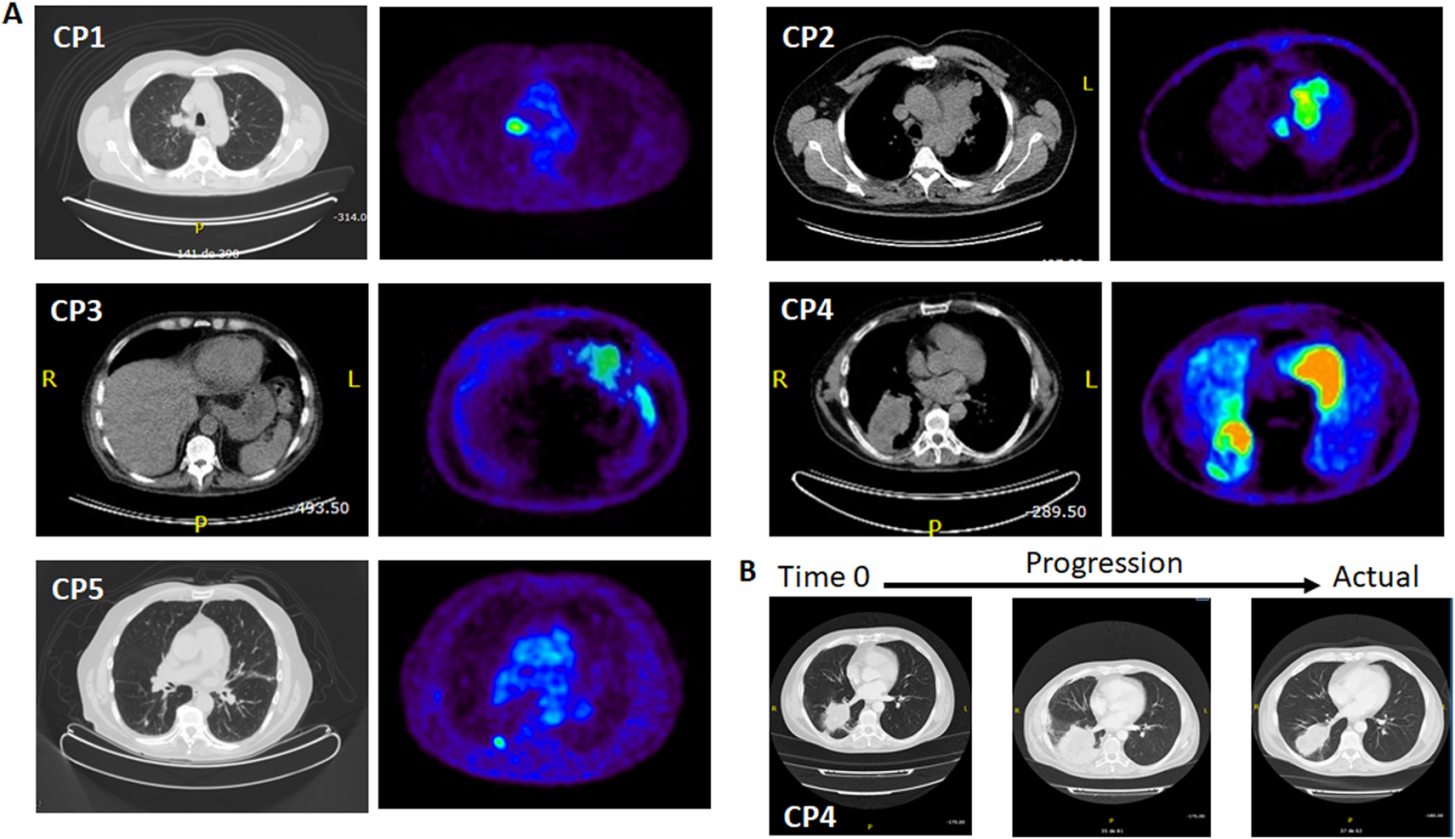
Computed tomography Positron emission tomography scanners. a Cross-sectional CT and PET-CT scans corresponding to the maximum size of the primary tumor from five patients. All patients are in stage IV (metastatic) but with different extensions and size of the primary tumor. CP1, adenocarcinoma patient with a non-bulky primary tumor, but with a very wide metastatic extension (subcutaneous); CP2 and CP3, CA patients with large primary tumors (bulky) but with limited extensions of metastasis; CP4, a priori, patient with a large primary tumor (bulky) with limited extension of metastasis (b); CP5, patient with a non-bulky primary tumor with limited extension of metastasis. b CT scan cross-sections showing the tumor progression of CP4 with treatment
